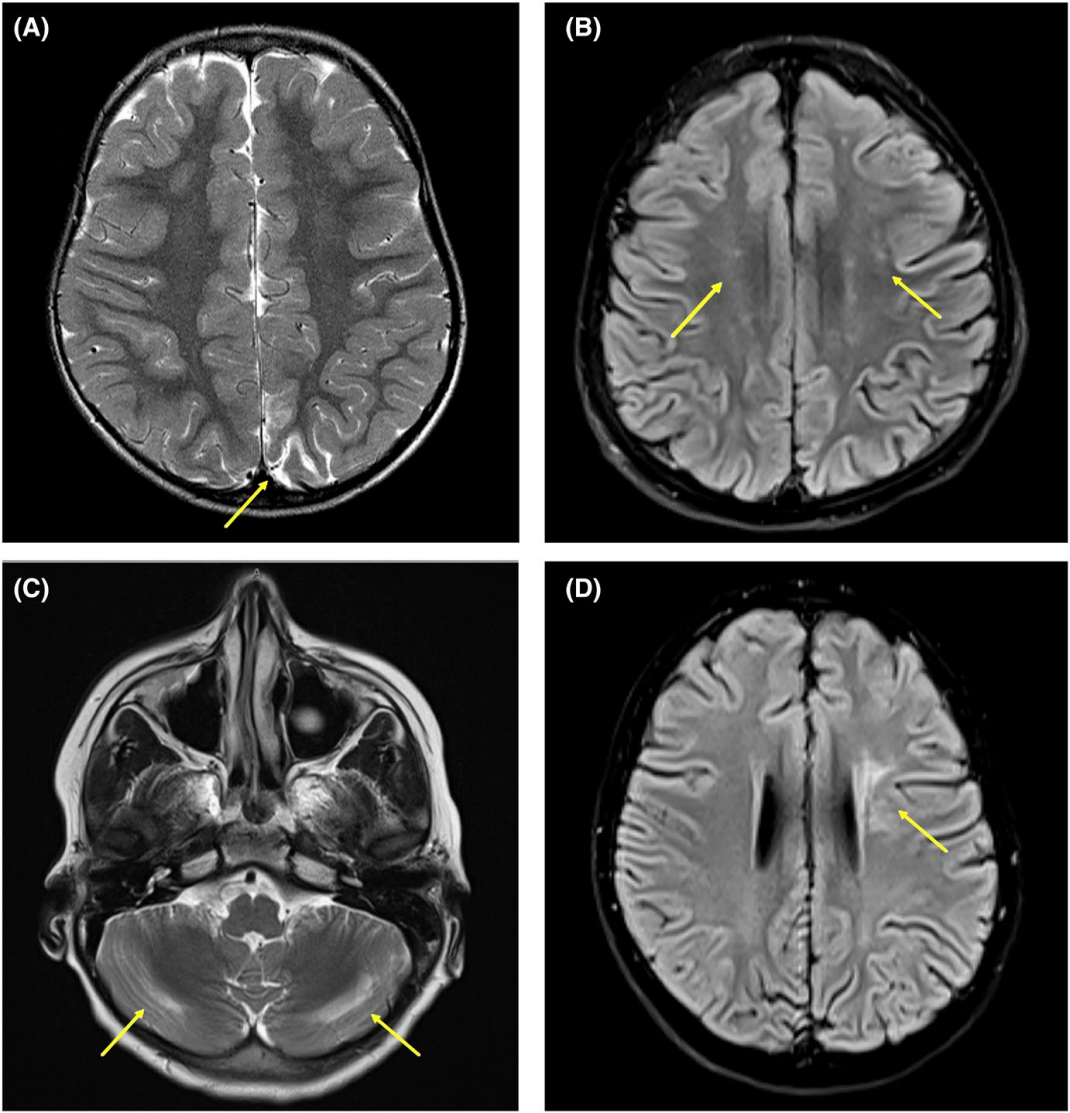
Neuroimaging Findings (Findings depicted by arrows). (A) Case 1: Axial T2. Focal cortical tissue loss at the posteromedial left cerebral hemisphere centered on the parieto‐occipital sulcus. (B) Case 2: Coronal FLAIR. Patchy FLAIR hyperintensities in the subcortical white matter. (C) Case 3: Axial T2. ↑T2 intensities in bilateral cerebellar hemispheres. (D) Case 4: Axial FLAIR. Increased FLAIR signal of the left frontal periventricular subcortical white matter. Cases 3 and 4 had symptomatic MTX neurotoxicity and increased FLAIR signals may reflect residual MTX neurotoxicity. Increased white matter intensities in Case 2 may reflect residual asymptomatic MTX neurotoxicity.
